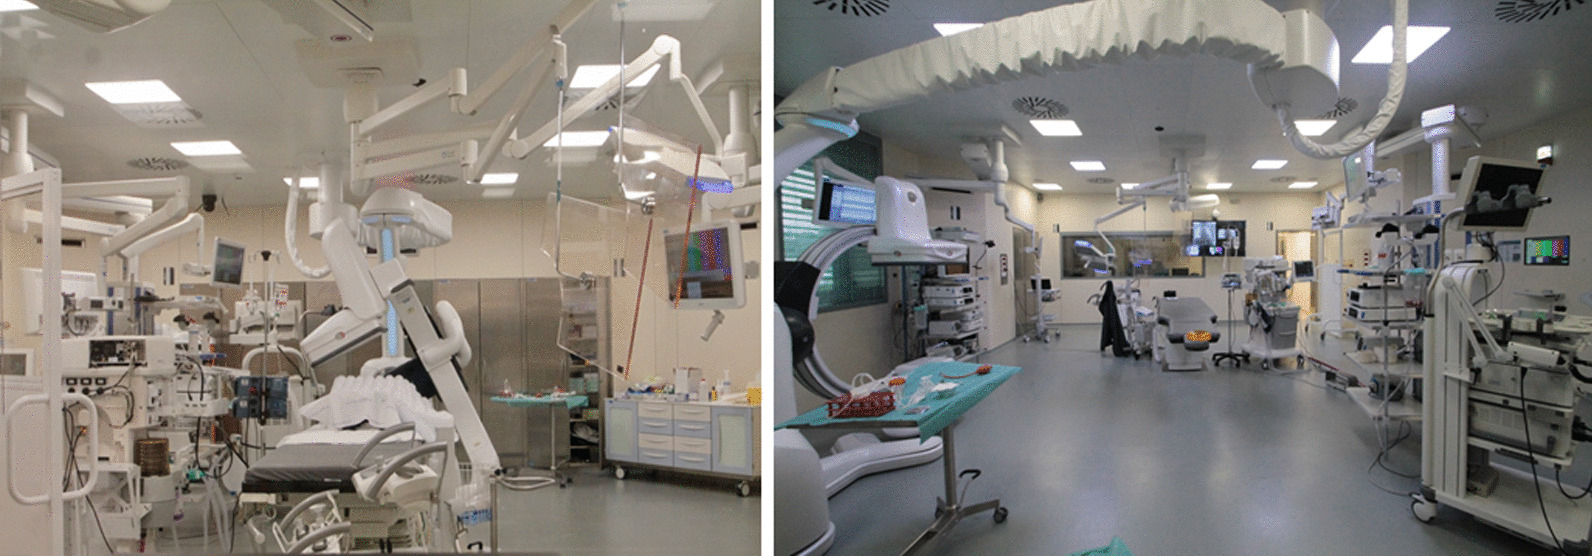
The hybrid operating theatre. The hybrid operating theatre with the instrumentation for the CBCT-guided bronchoscopy
